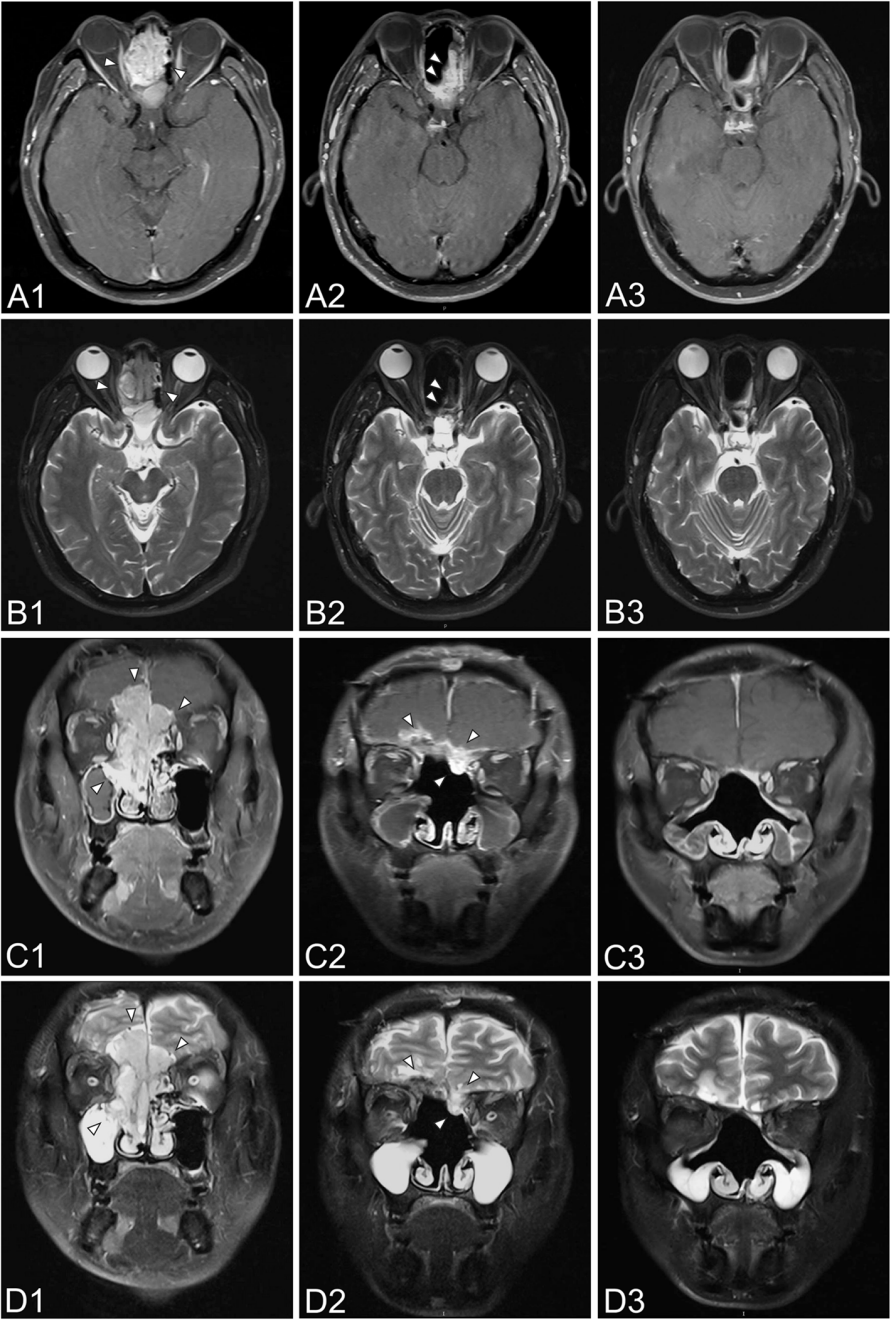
Comparison of MRI before and after treatment for a 38-year-old patient with ENB (stage of C) who received surgery plus postoperative radiation. (A) and (B) refer to axial MRI, and (C) and (D) refer to coronal MRI. 1, 2, and 3 refer to MRI before treatment, after surgery, and after radiotherapy, respectively. MRI before treatment revealed a large mass on bilateral nasal cavity and ethmoid sinus, which invaded the extraconal fat of the right orbit (A1 arrowhead, B1 arrowhead). The tumor extended to right maxillary sinus and sphenoid sinus, bilateral anterior skull base and frontal lobe (C1 arrowhead, D1 arrowhead). After surgery, residual tumor was observed at the site of ethmoid sinus (A2 arrowhead, B2 arrowhead), bilateral anterior skull base and frontal lobe (C2 arrowhead, D2 arrowhead). After postoperative radiation, the residual tumor disappeared
